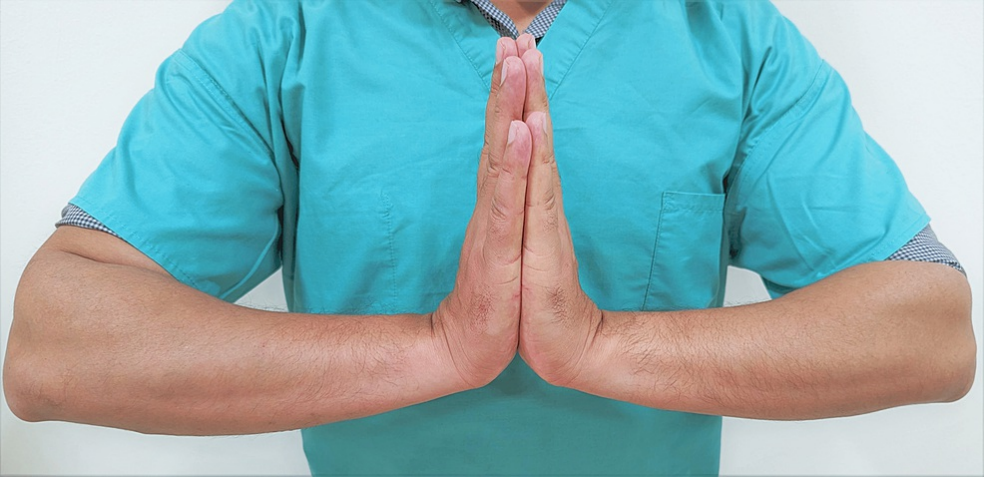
Reverse Phalen's test This is an original image from the ultrasound lab department of the American University of Antigua
